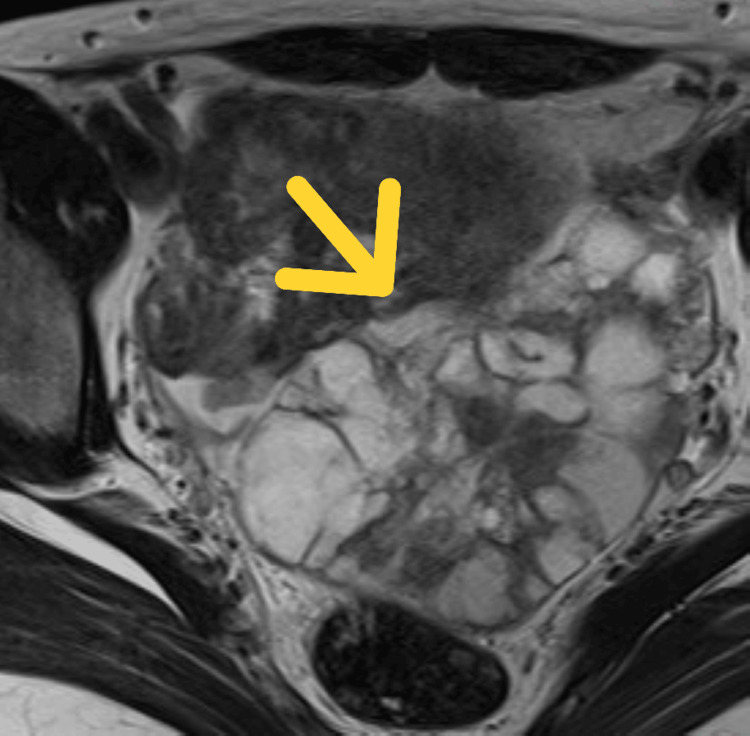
Axial T2-weighted MRI showing the ovarian mass Axial T2-weighted MRI demonstrating a 106 × 68 mm multilocular cystic ovarian mass with internal septations and heterogeneous high signal intensity, raising suspicion for malignancy. The arrow indicates the ovarian mass.
